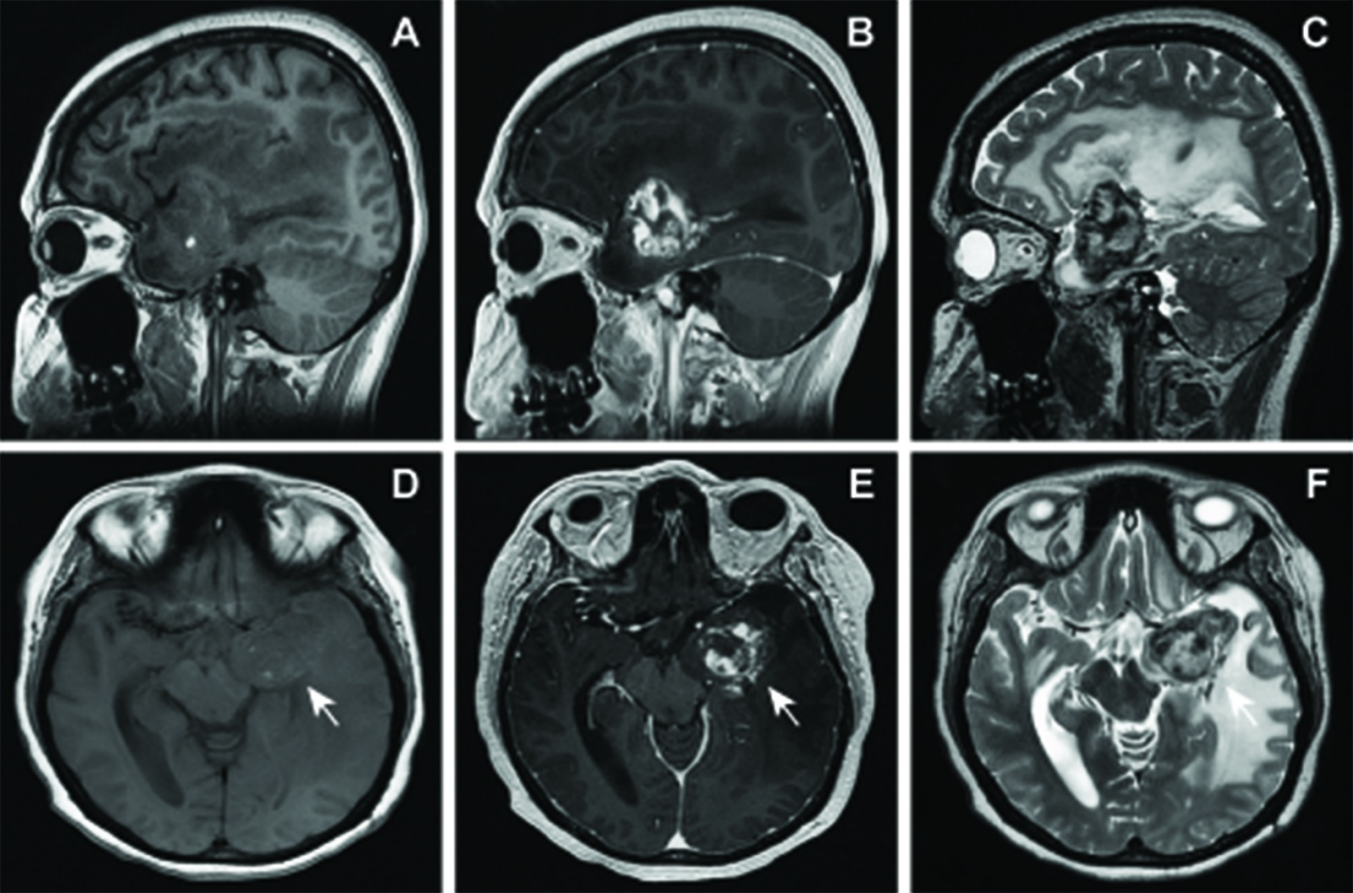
Preoperative MRI obtained 15.5 years following radiosurgery showing interval expansion and hemorrhage of radiation-induced lesion. Sagittal and axial T1 weighted without constrast (A,D), with gadolinium contrast (B, E), and T2-weighted (C, F) images demonstrating progressive enlargement of lesion within left parahippocampal gyrus with small amount of intrinsic T1 signal, peripheral enhancement and extensive surrounding edema. The lesion exerts extensive mass effect with effacement of the left lateral ventricle, 7 mm of midline shift and herniation of the left uncus leading to compression of the left cerebral peduncle and midbrain. Arrows, region of interest.
